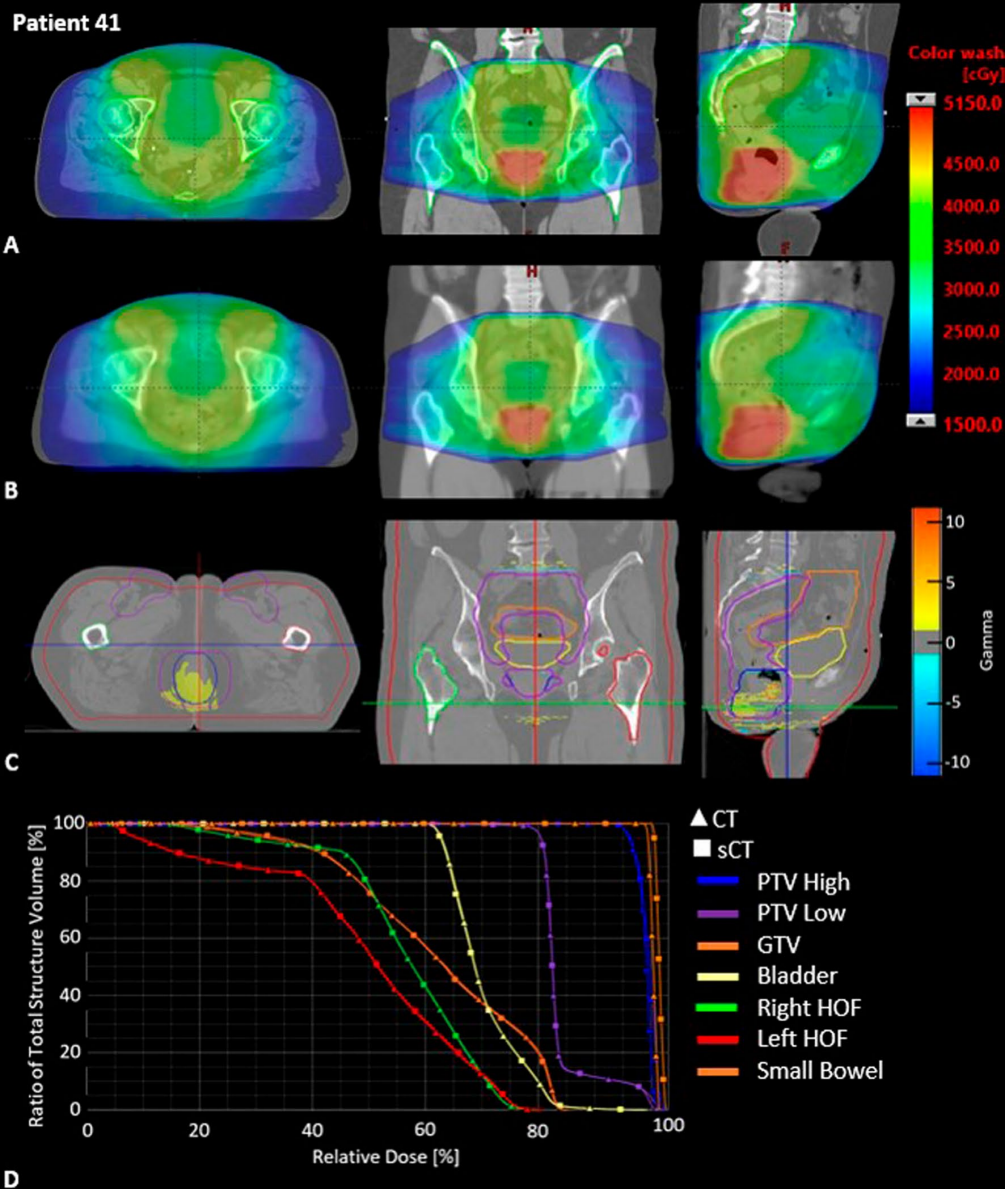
Results for patient 41 (worst performing gamma). Row A Original patient CT scan with dose overlayed. Row B sCT with dose overlayed. Row C CT scan and critical structure outlines with Gamma map overlayed (1%/1 mm) colour wash showing regions which do not meet the gamma pass rate (values between 1 and − 1 not displayed). Row D Dose volume histogram results
